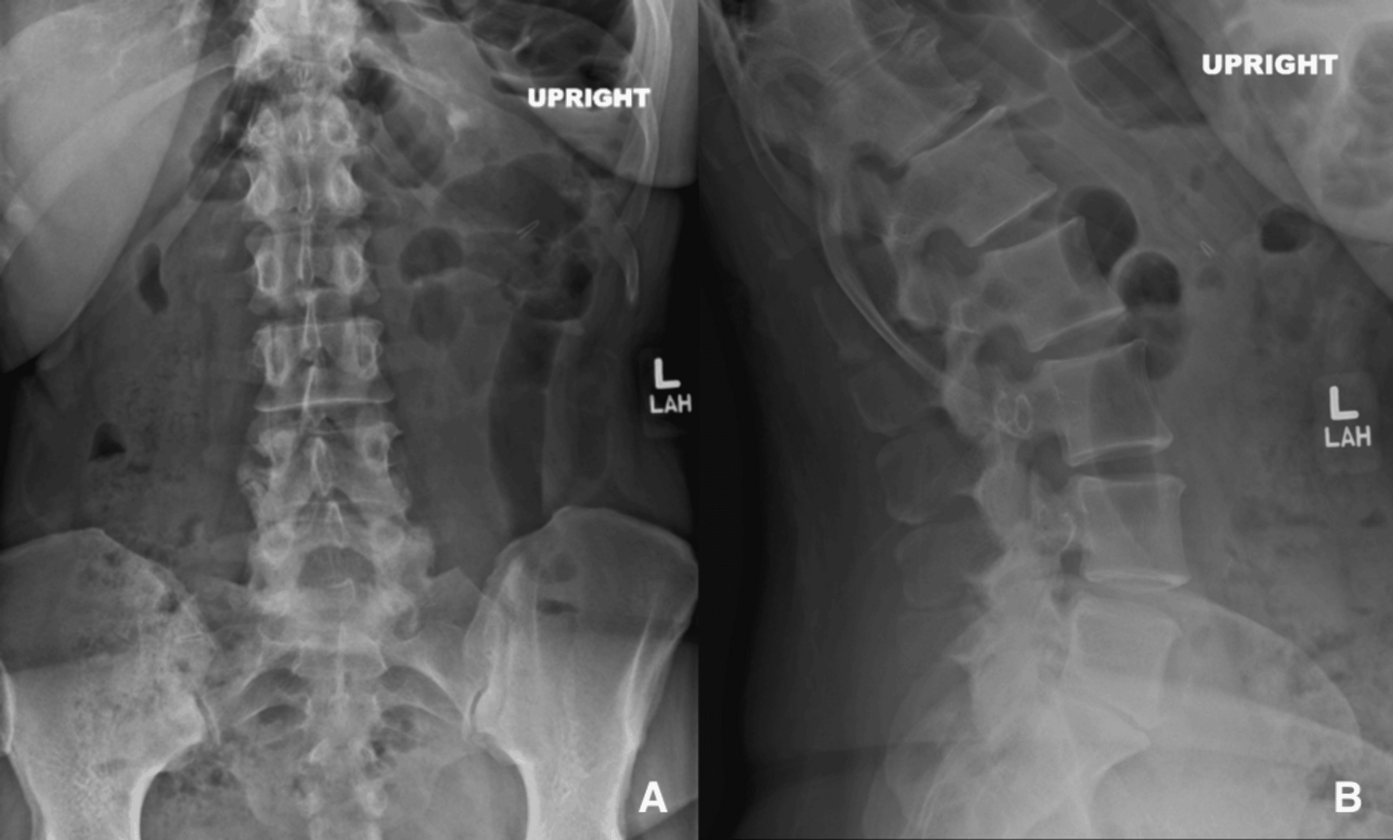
Radiographs of the lumbar spine. (A) anterior-posterior view; (B) extension view
